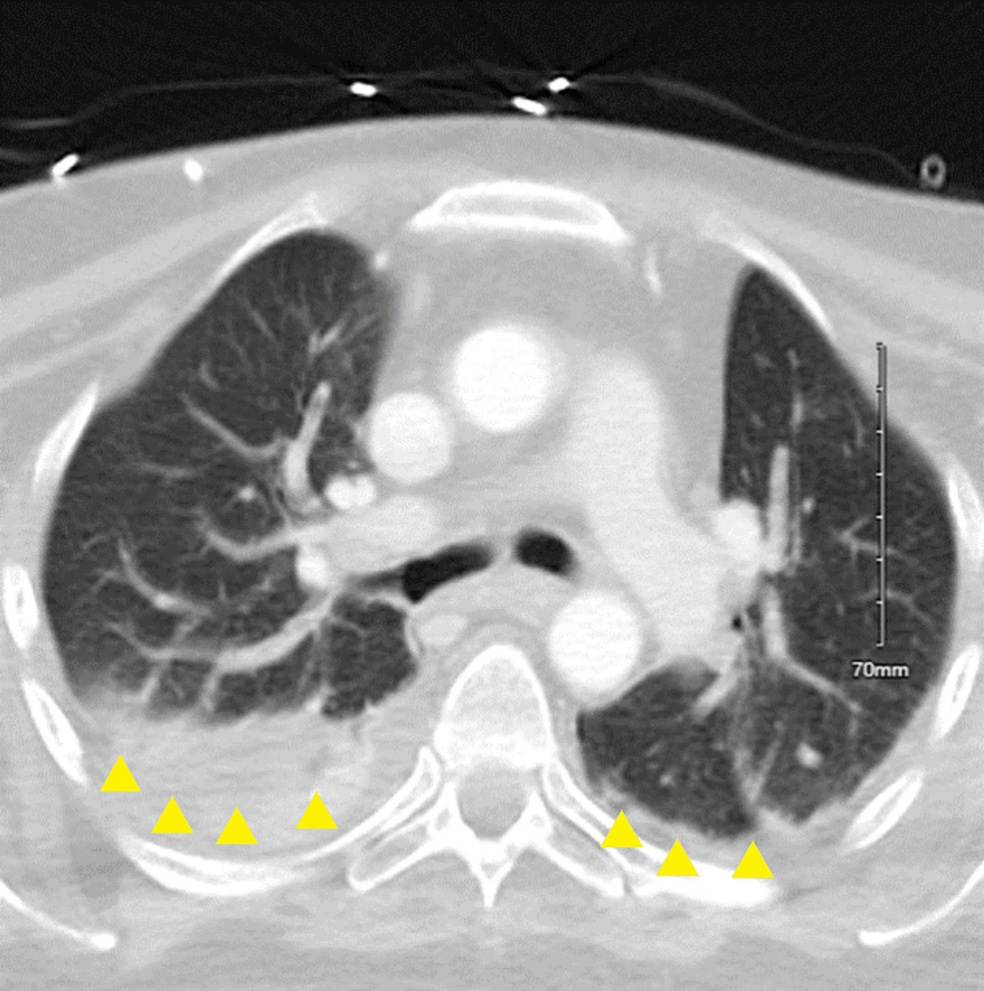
CT chest with IV contrast day 1 CT chest with IV contrast imaged on hospitalization day 1 with bilateral pleural effusion right worse than left. Bilateral lower lung airspace disease was identified.
